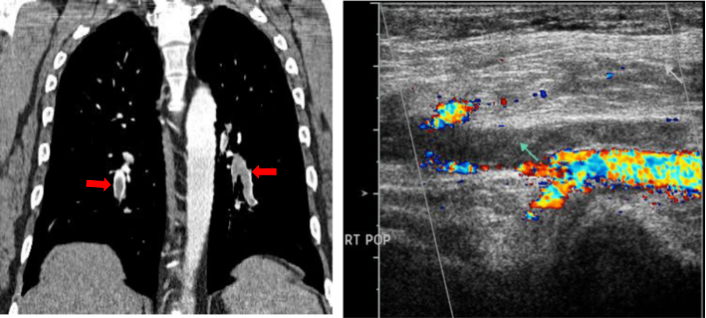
39 year old male with glioblastoma multiformae on Bevacizumab. A. Coronal CT demonstrates multiple bilateral pulmonary emboli (arrow). B. US Doppler of the right lower extremity demonstrates echogenic material completely filling the left popliteal vein (arrow) and extending to the proximal left posterior tibial vein consistent with Deep Venous Thrombosis.
